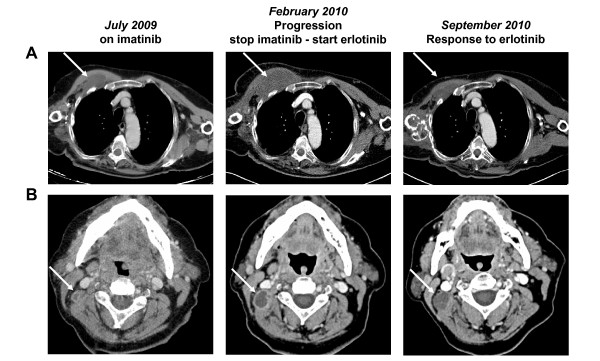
Cervico-thoracic CT scan showing response to erlotinib. Three different dates of treatment are shown: July 2009 (on imatinib), February 2010 (progression on imatinib; just before erlotinib), and September 2010: (response after 7 months of erlotinib). Two targets lesions are shown (arrows): the right pectoral lesion (A), and the right cervical adenopathy (B): both progressed between July 2009 and February 2010 during imatinib treatment, and responded to erlotinib between February and September 2010: regression in size of the pectoral lesion (A), and disappearance of the peripheral contrast enhancement for the adenopathy (B).
